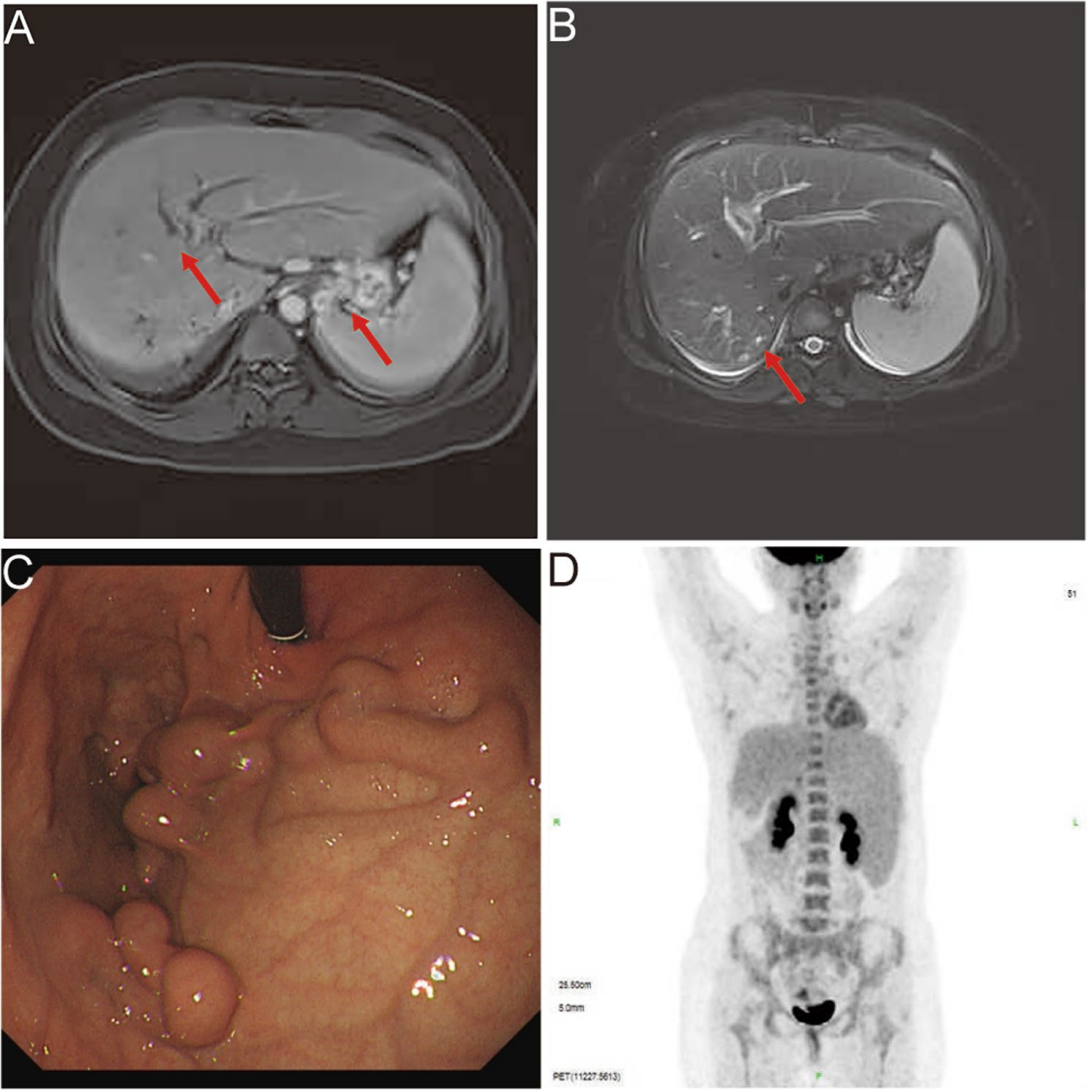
Imaging findings of the patient. (A-B) MRI imaging revealed dilated intrahepatic bile duct dilatation, gastric fundic varices, an enlarged spleen and small cyst in the kidney (arrow). (C) Upper gastrointestinal endoscopy revealed gastric fundic varices. (D) PET/CT imaging revealed slightly enlarged liver, hyperplastic lymph nodes around the portal vein, giant spleen with no increase in FDG metabolism and multiple varices in the fundic and retroperitoneum
